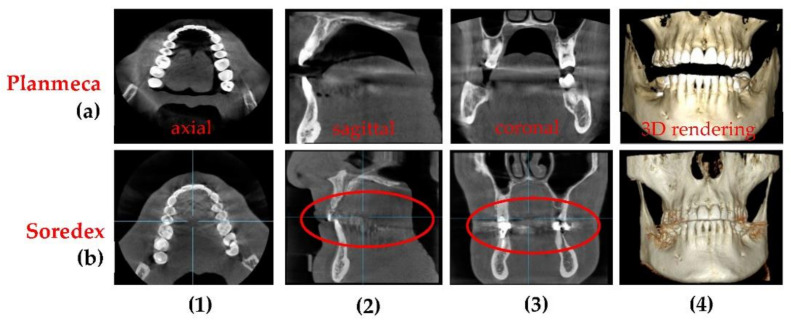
3D CBCT performed on the same patient (C.C, male, 48 years old) with (a) the Planmeca unit and with (b) the Soredex unit. Notations: (1) axial, (2) sagittal, (3) coronal, and (4) 3D rendering.
